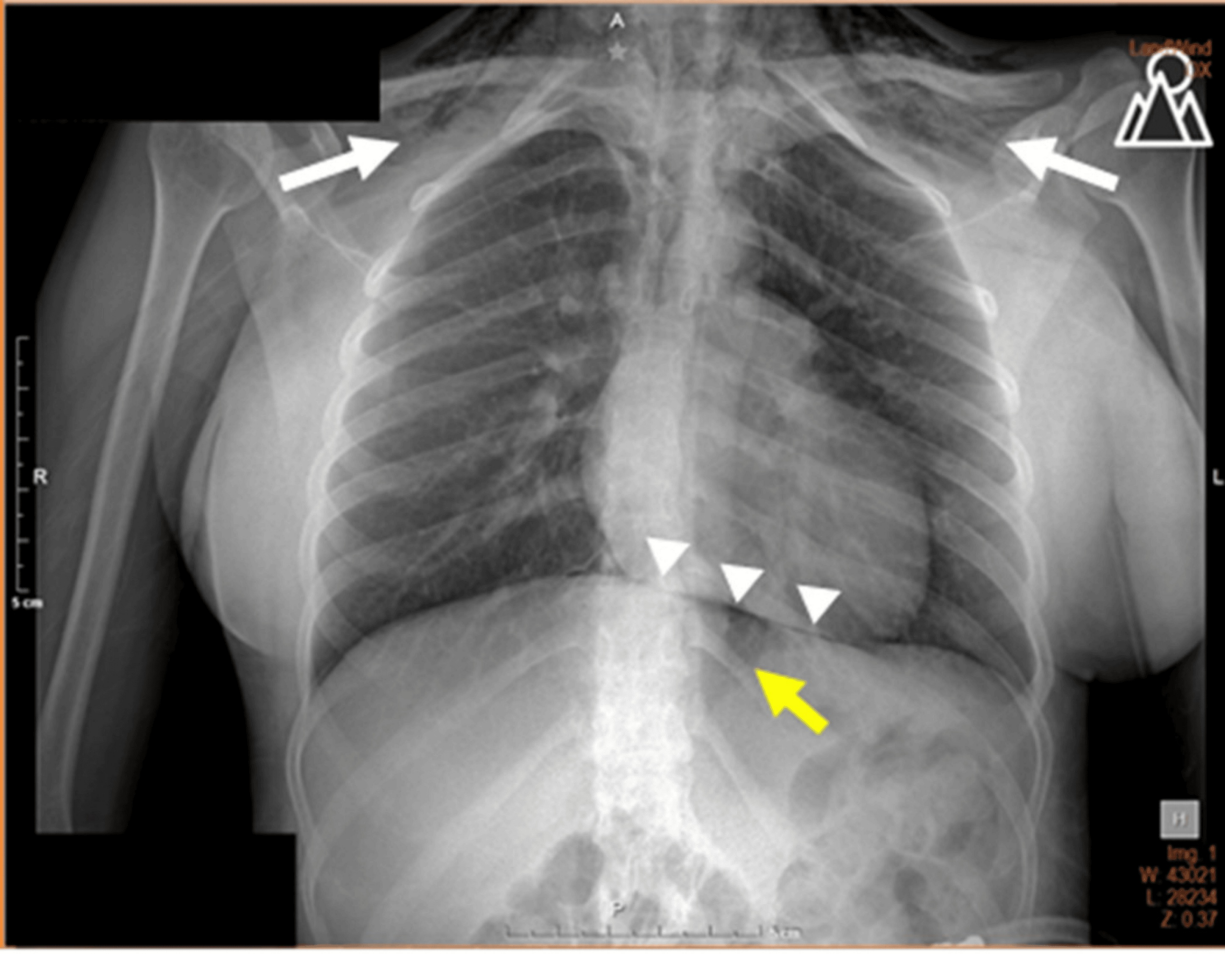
Anteroposterior chest X-ray on day zero The image shows subcutaneous emphysema in the clavicular regions extending to the neck (white arrows), with radiographic signs of pneumomediastinum, including Naclerio’s V sign (yellow arrow) and the continuous diaphragm sign (white arrowheads).
